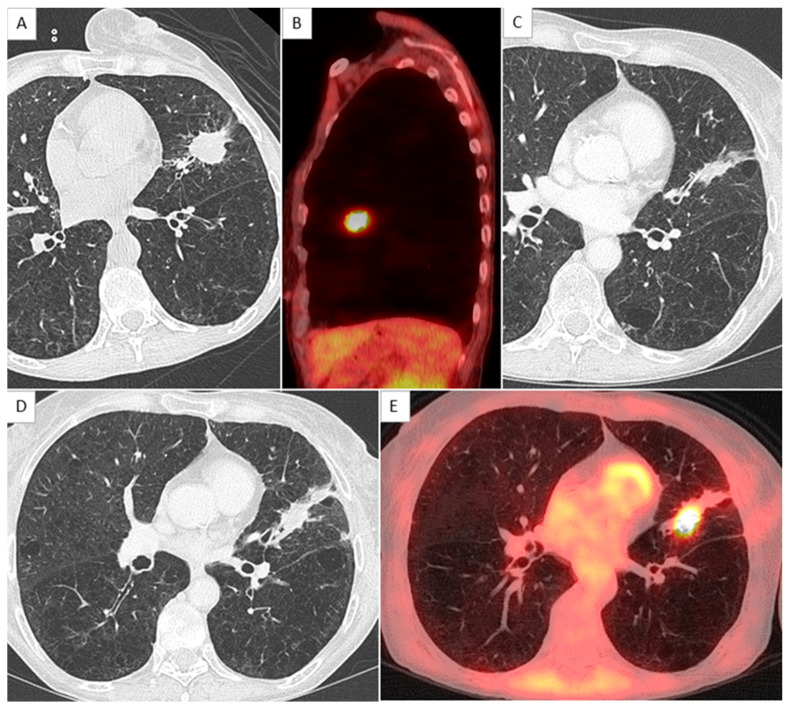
Example of local relapse in a female patient with score at 3. (A,B) show a lingular mass on axial chest CT and sagittal merge PET and CT, diagnosed after sampling as an adenocarcinoma, with hypermetabolism quantified as SUVmax = 8.0. (C) shows the scar 1 year after the treatment, and (D,E) show a local recurrence 2 years after radiation therapy.
